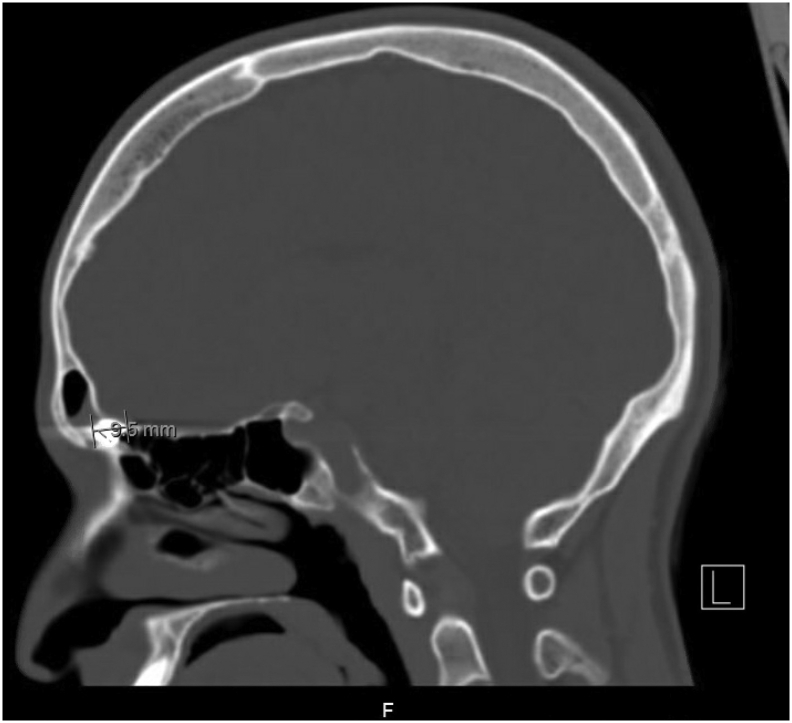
CT showing a foreign object in the frontal sinus. Parasagittal CT of the paranasal sinuses on bone window showing 9.5 mm wide foreign object (air pellet) in the inferior aspect of the frontal sinus.
